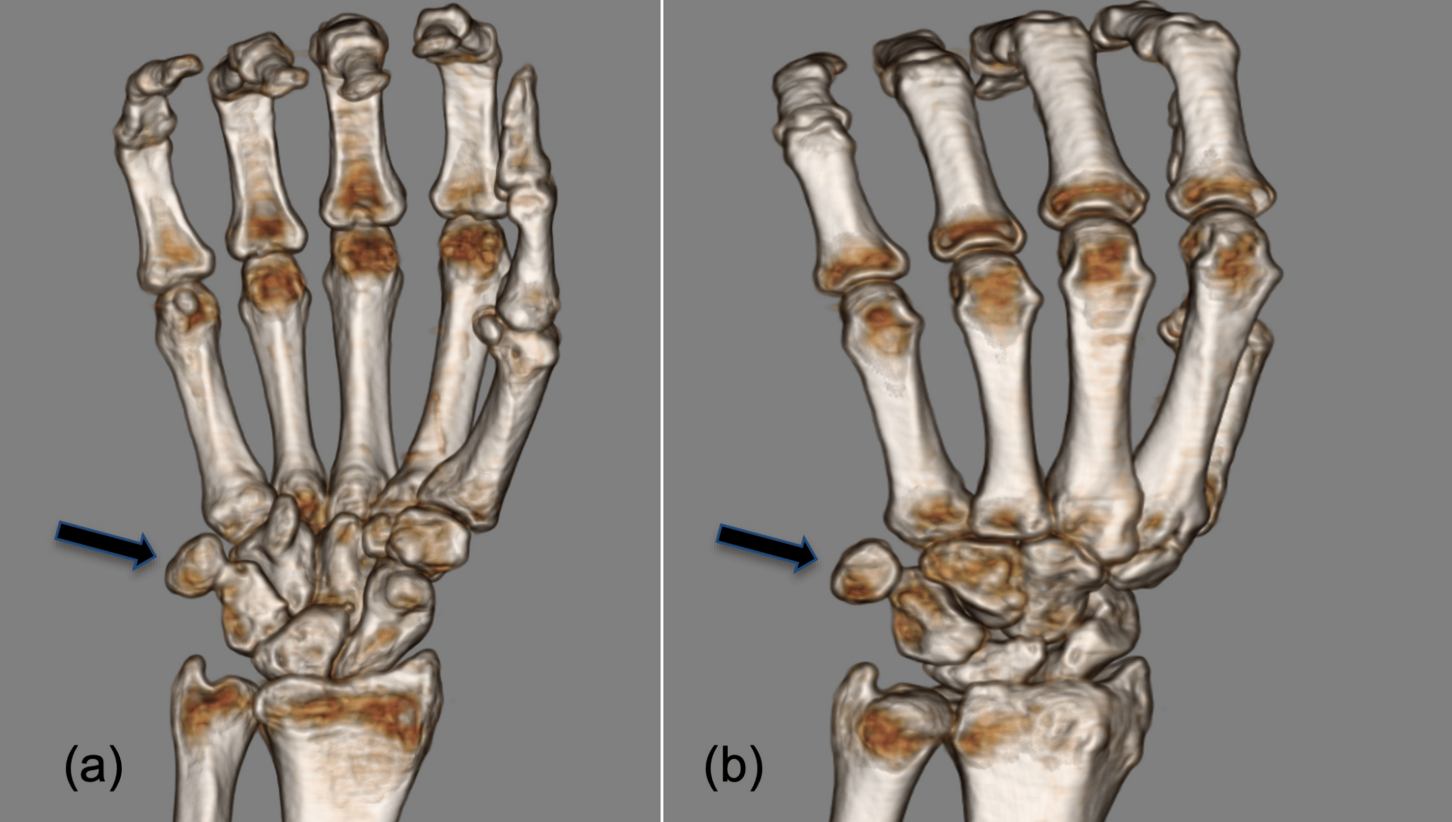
Coronal plane 3D reconstructed images (a, b) of a CT scan displaying dorsal ulnar displacement of the pisiform
